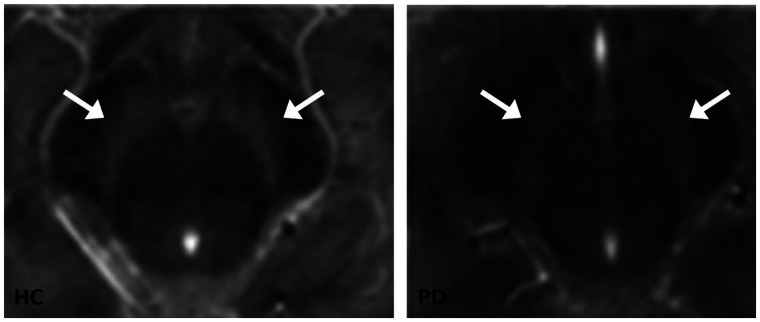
Transversal images of neuromelanin-sensitive MRI scans of mesencephalon. Substantia nigra is visible as hyperintense area next to cerebral peduncles. Left panel shows example of neuromelanin-sensitive MRI in healthy control, and right panel shows example of patient with PD. Loss of signal can be seen in substantia nigra in PD patient. Arrows point to substantia nigra. (Reprinted from (74).)
